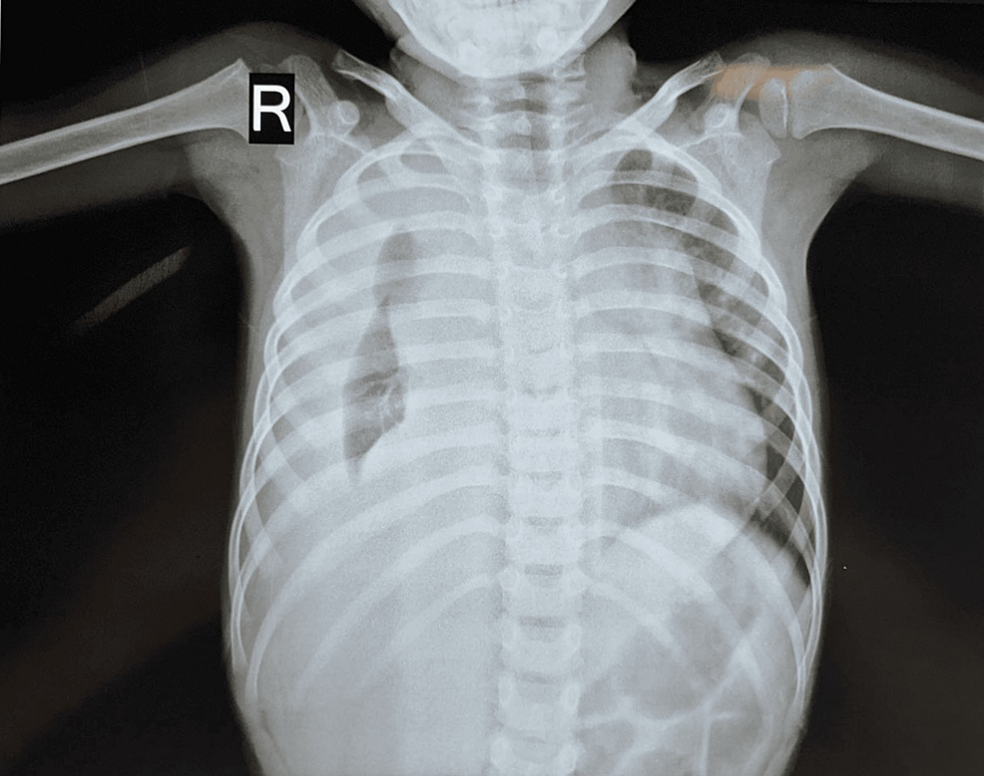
Initial chest X-ray showing diffused opacity
